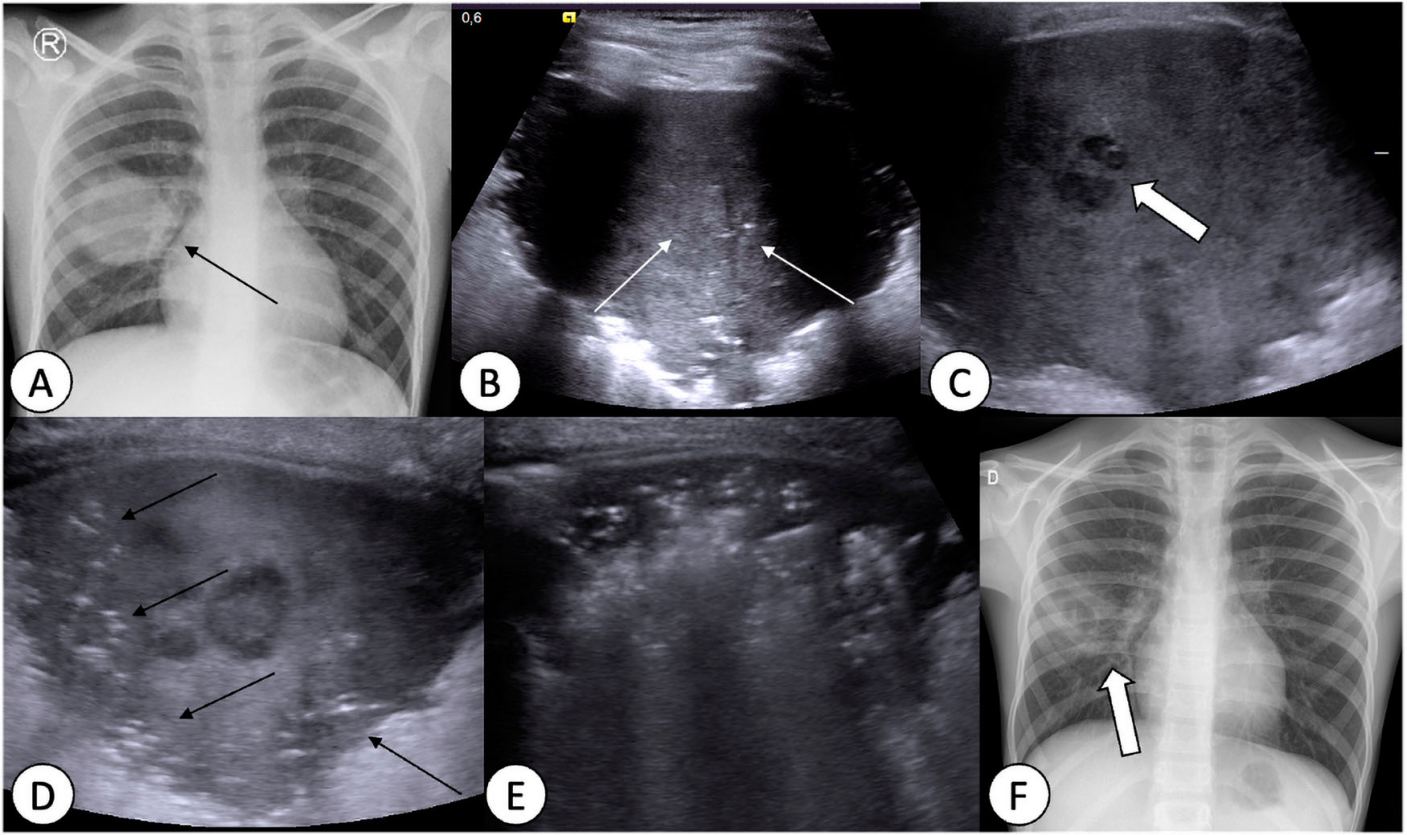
Clinical progression of round pneumonia in an 11-year-old boy. A Chest radiograph (CR) on admission revealing a round opacity (arrow) within the right hemithorax. B Lung ultrasound (LUS) shows oval-shaped subpleural consolidation with mild central increase in echogenicity (white arrows) related to the pre-necrotic stage of round pneumonia. C Two days later, LUS revealing distinct small hypoechogenic zones of necrosis (thick arrow). D Reaeration starting at the periphery of consolidation (thin black arrows), with persistent central necrosis observed after 3 days. E One week later, lung consolidation significantly reduced in size with diffuse reaeration and absence of detectable necrotic zones. F Follow-up CR confirming significant regression of pneumonia
